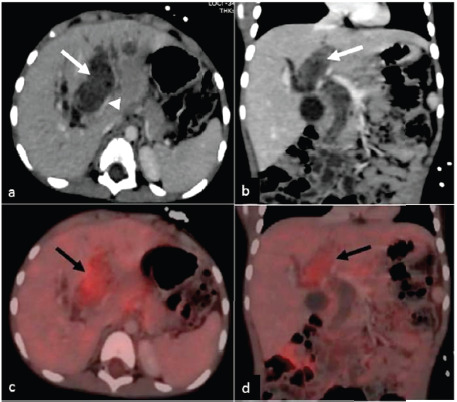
Post contrast computed tomography axial (a) and coronal (b) image demonstrating a mild enhancing heterogeneous mass within the common bile duct and left hepatic duct(arrow) causing compression of left portal vein seen(arrowhead). Axial (c) and coronal (d) PET CT showing mild metabolic activity within the soft tissue mass (black arrow).
